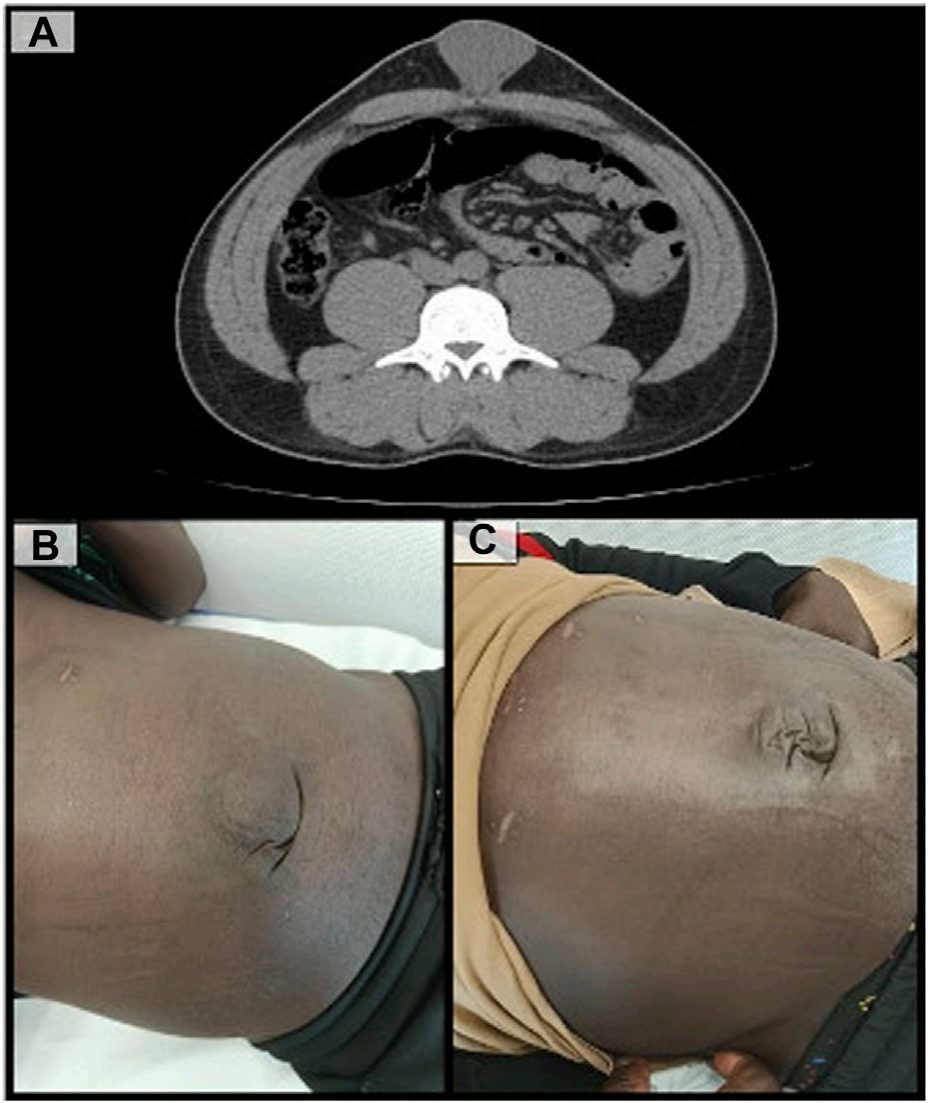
(A): CT scan showing corrected diastasis recti and a significant postoperative seroma; (B): Patient follow-up at one month postoperatively; (C): Patient follow-up at three months postoperatively.
